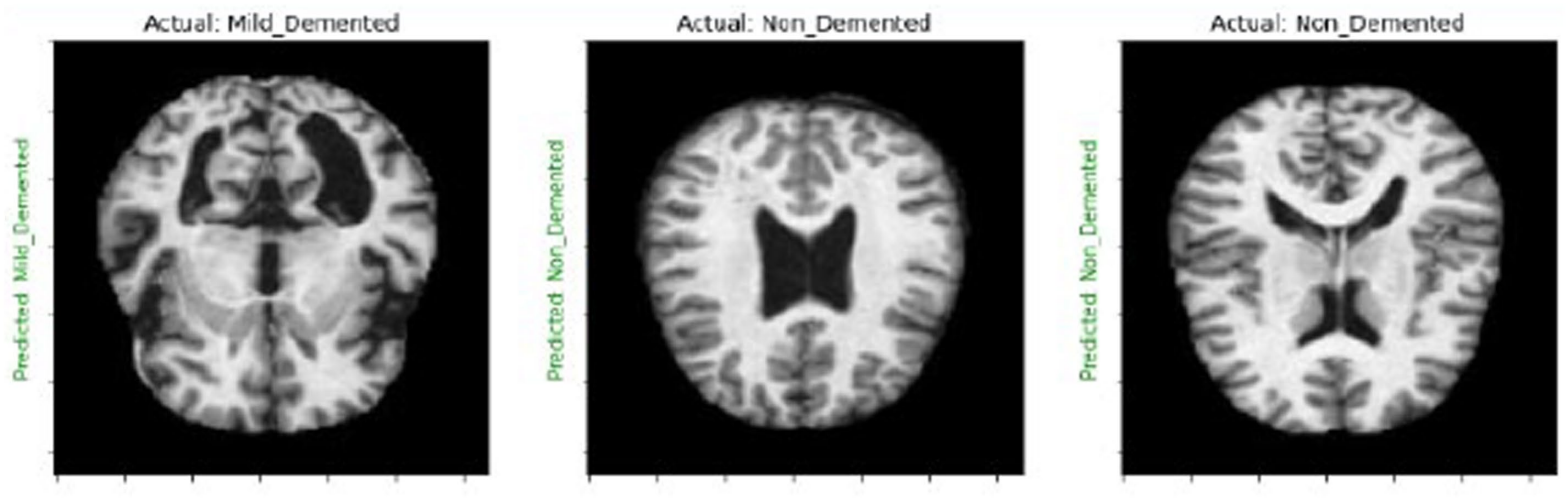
MRI images labeling after diagnosis of the disease.
